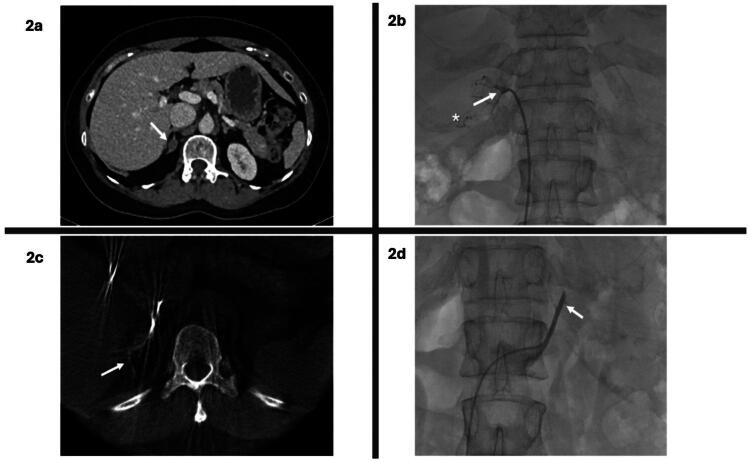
Multimodal imaging in adrenal vein sampling. (a) Computed tomography scan with IV contrast – venous phase showing right adrenal adenoma (arrow). (b) Right adrenal vein cannulation at the level of T12 confirmed with contrast enhancement of the right adrenal gland (arrow). Note emissary vein (asterisk). (c) DynaCT demonstrating enhancement of the right adrenal vein (arrow) during the AVS procedure. (d) the left adrenal vein common trunk (arrow) cannulation during AVS procedure.
